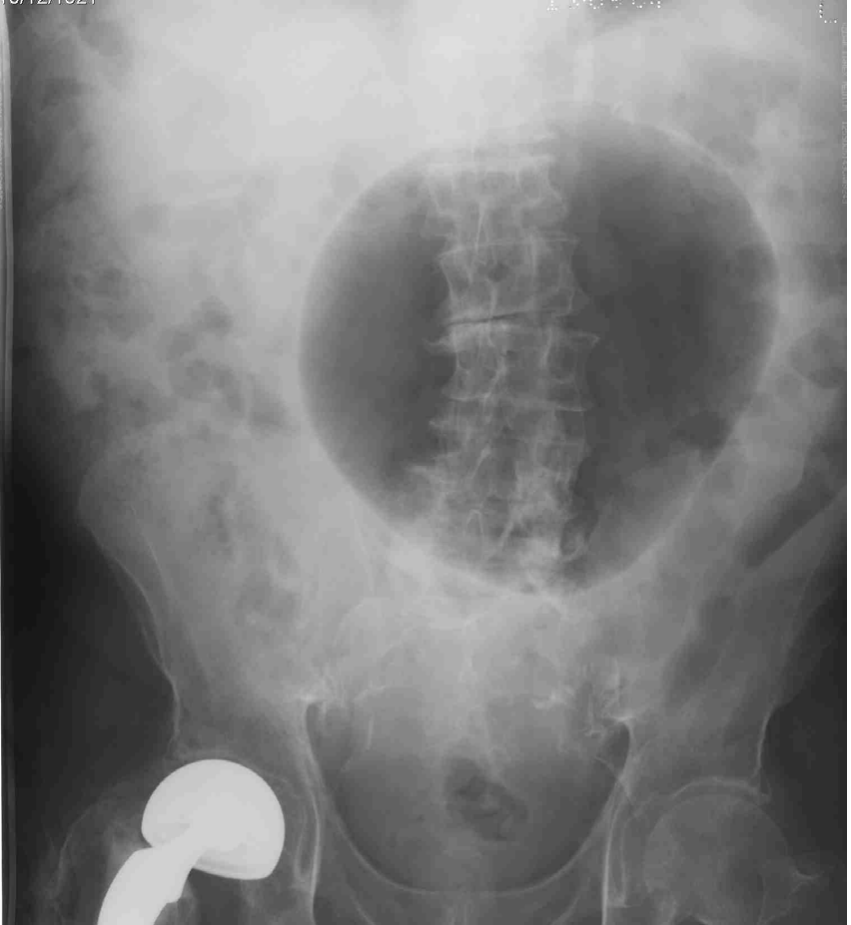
Plain abdominal radiograph showing homogenous radiolucency that is smoothly marginated.
